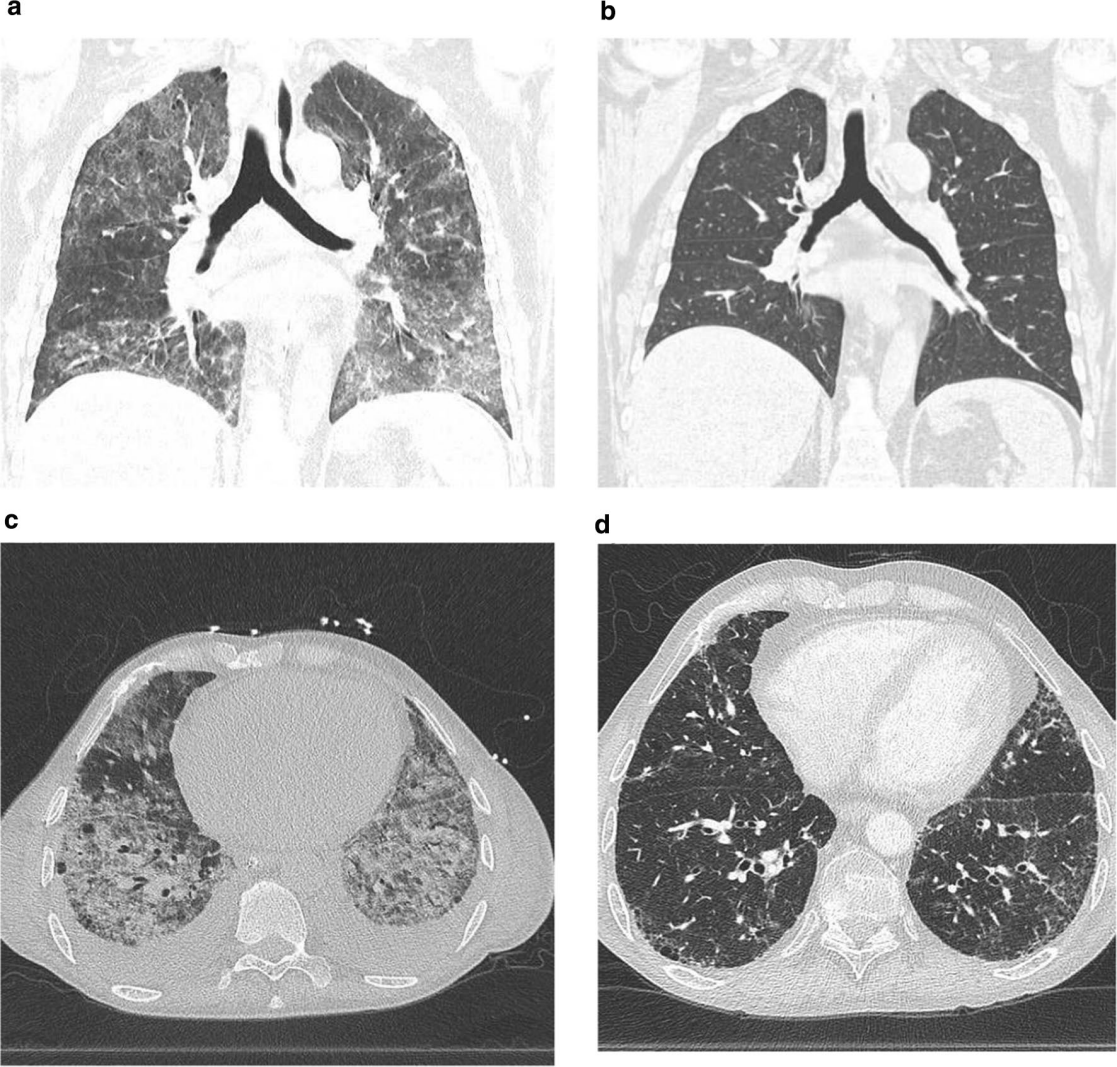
CT scans of two patients before cyclophosphamide treatment and after cyclophosphamide treatment. Coronal CT scan: case 8 (SLE-like disease) before cyclophosphamide treatment (a) and after cyclophosphamide treatment (b). Axial CT scan: case 10 (MCTD-ILD) before cyclophosphamide treatment (c) and after cyclophosphamide treatment (d)
